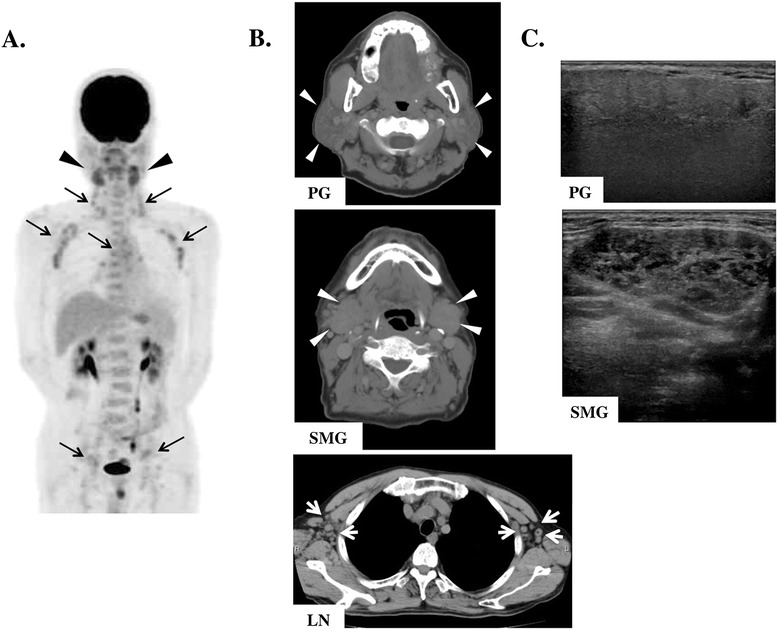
Imaging findings before treatment. (A) Fluorodeoxyglucose positron emission tomography (FDG-PET) indicating abnormal multiple accumulations in the submandibular glands (SMGs) (white arrowheads) and in several systemic lymph nodes (black arrowheads). (B) Computed tomography (CT) findings indicating swelling of the parotid glands (PGs) (white arrowheads), SMGs (white arrowheads), and generalized lymph nodes (LNs) (white arrows). (C) Sonographic images indicating bilateral nodal hypoechoic areas in the SMGs.
